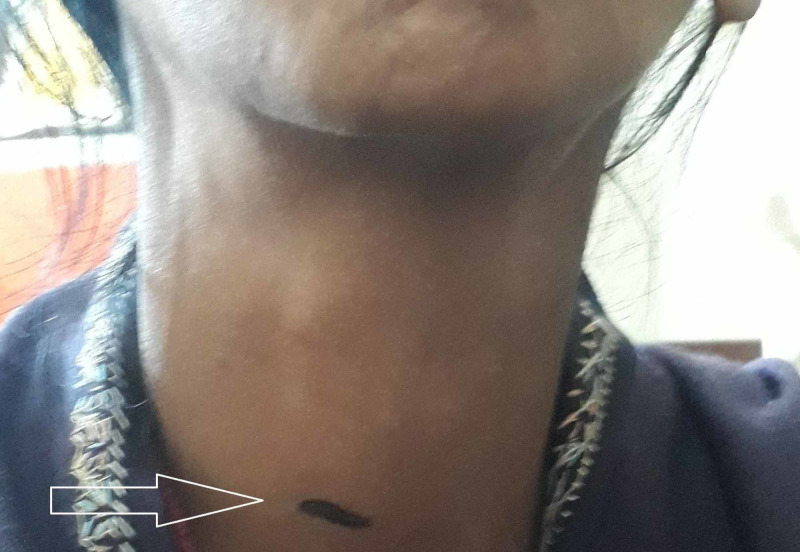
Preoperative skin marking of right superior parathyroid gland, as confirmed by ultrasonography (white arrow).
